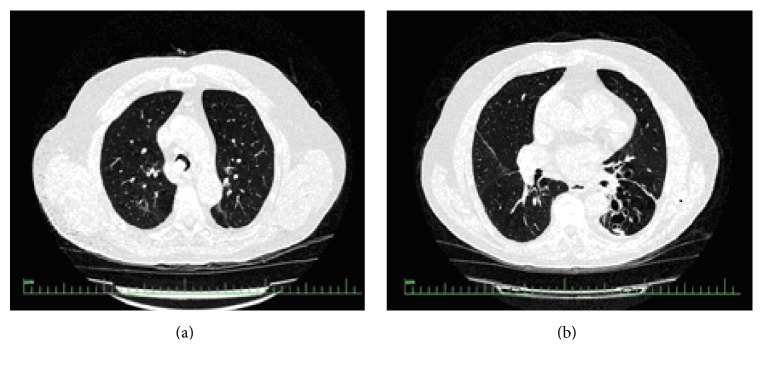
Computed tomography scan revealed the mass in the lumen of trachea (a) and cystic bronchiectasis on left lower lobe (b).
